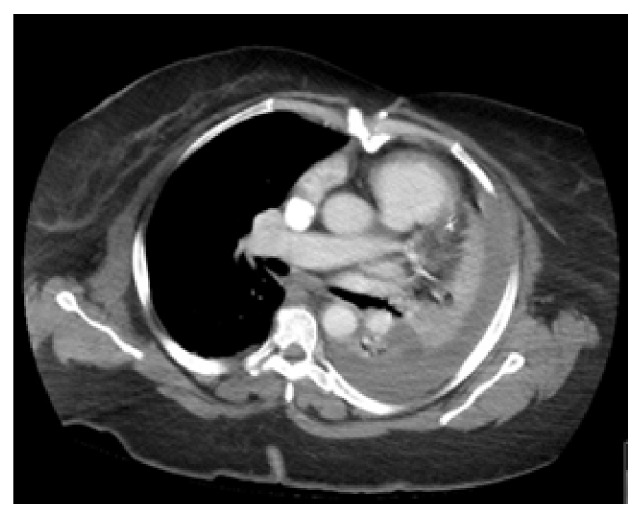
Chest CT with IV contrast after drainage of one liter. No vascular malformations, active bleeding, or other sources of hemothorax were identified. Left-sided pleural effusion was noted to have different Hounsfield unit when compared to the aorta and pulmonary artery.
